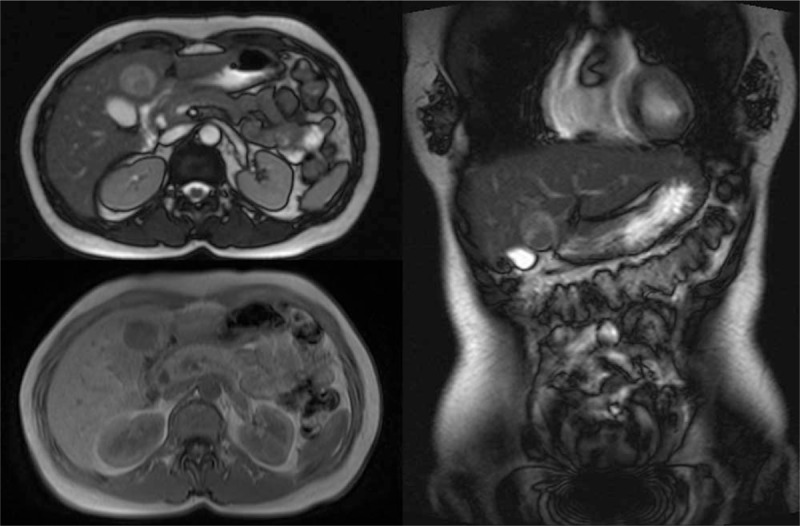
The magnetic resonance imaging (MRI) examination of the upper abdomen showed a well-defined, 30×29 mm^2^ approximately, a nearly-circular shadow in the IVb segment of the liver, with low signal intensity on T1W imaging and slightly high signal intensity on T2W imaging.
